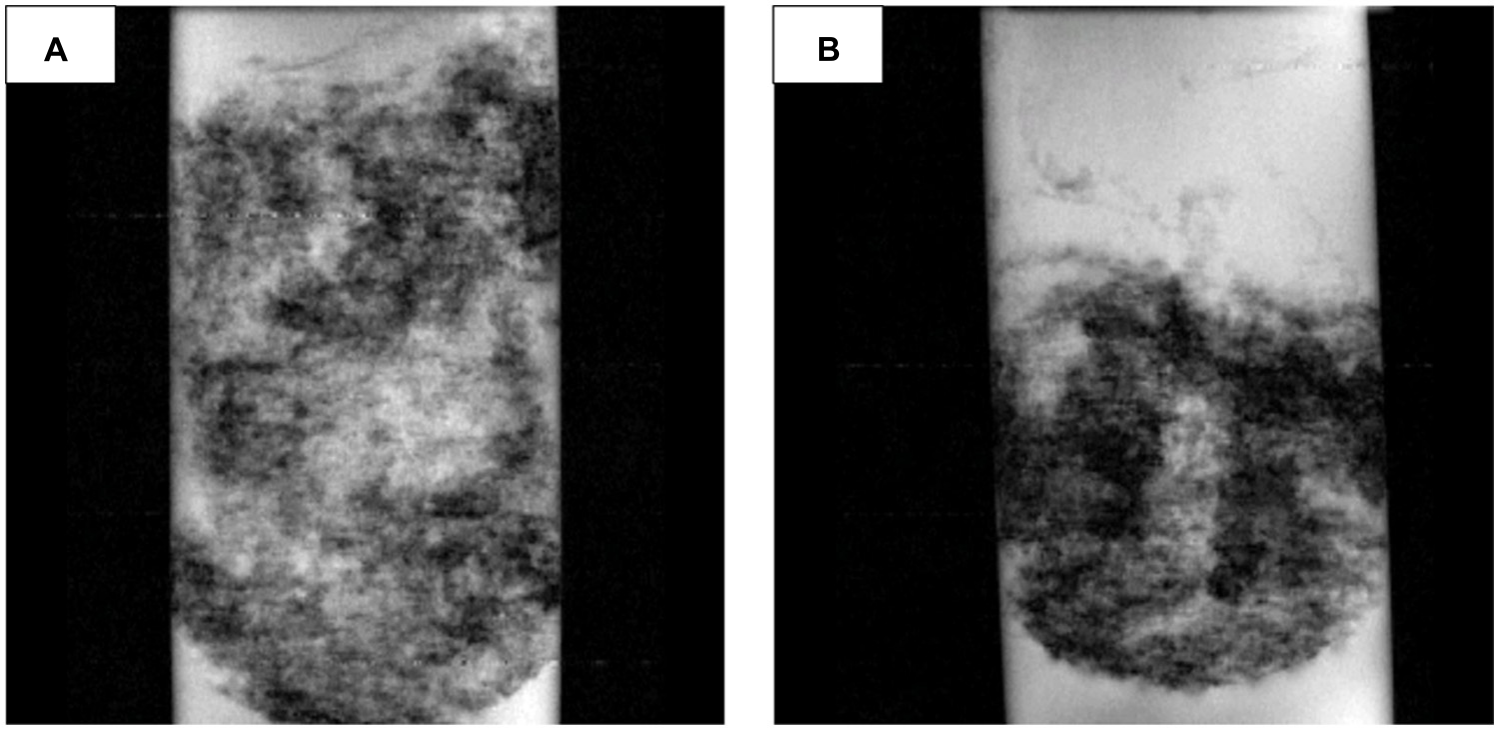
Representative nuclear magnetic resonance images (97.7 μm × 78.1 μm resolution, 5-mm slice) of (A) the CAT mat and (B) the AN mat.
